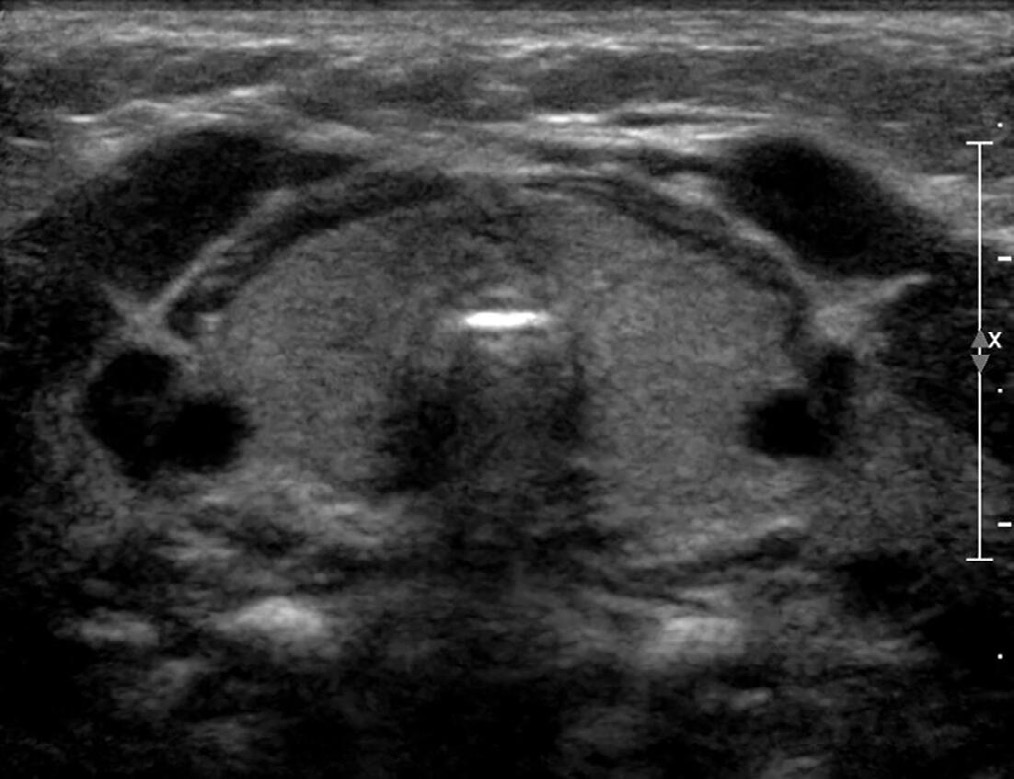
Ultrasound of infant with compound heterozygous *DUOX.2* mutation (patient P7, Table 1), showing loss of normal curves of anterior margin of thyroid. Objective measurement of the gland at 1.75 ml falls within normal limits.
